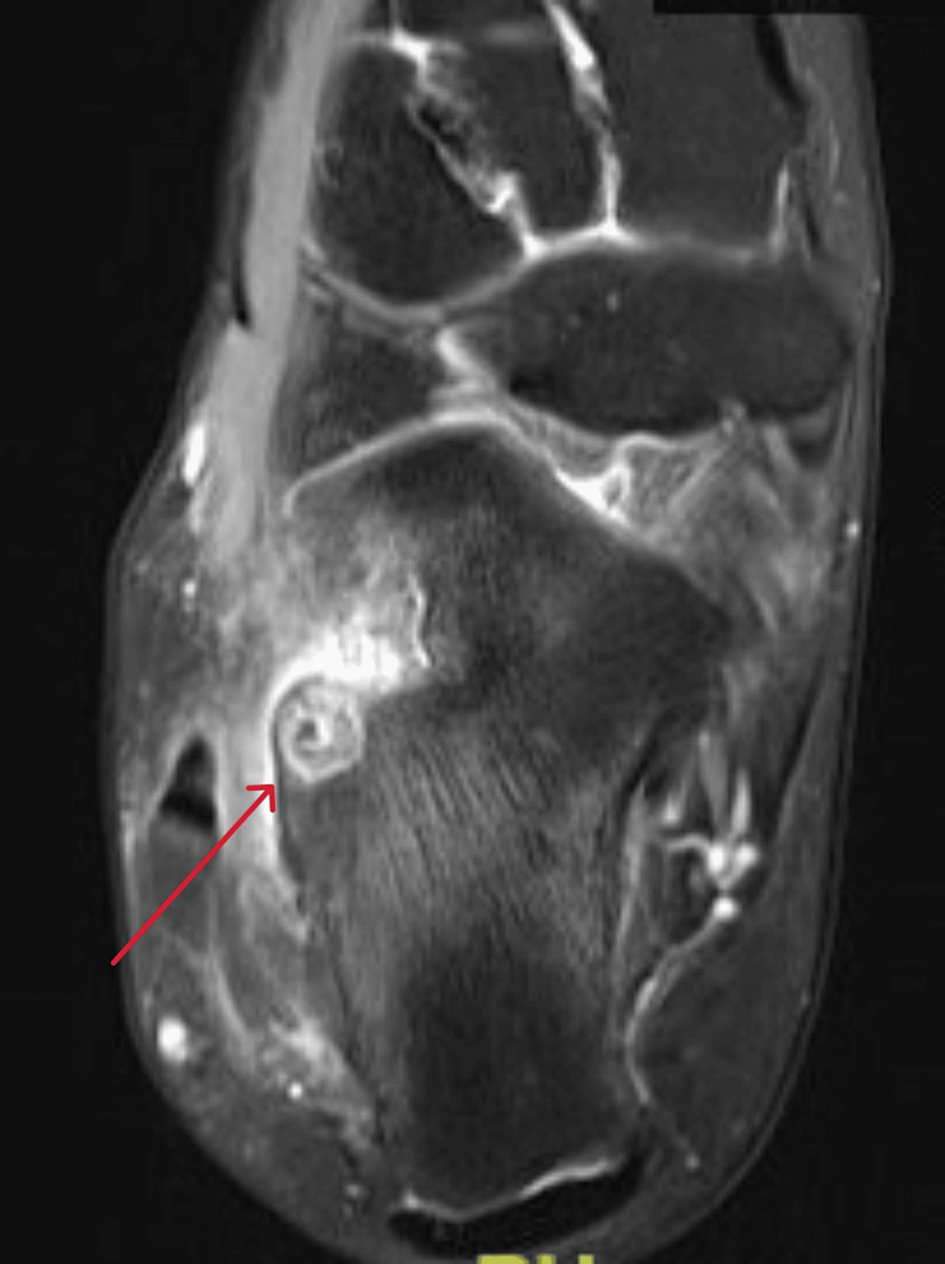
Axial view MRI T2 window of the right ankle at the time of presentation MRI: magnetic resonance imaging
